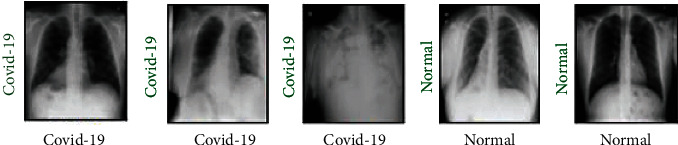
Training images for X-ray images of COVID-19 and normal.
